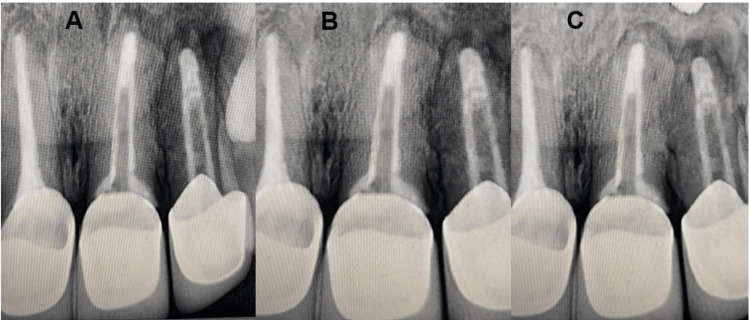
Radiographic follow-up (A) Three months follow-up. (B) Six months follow-up. (C) Nine months follow-up
